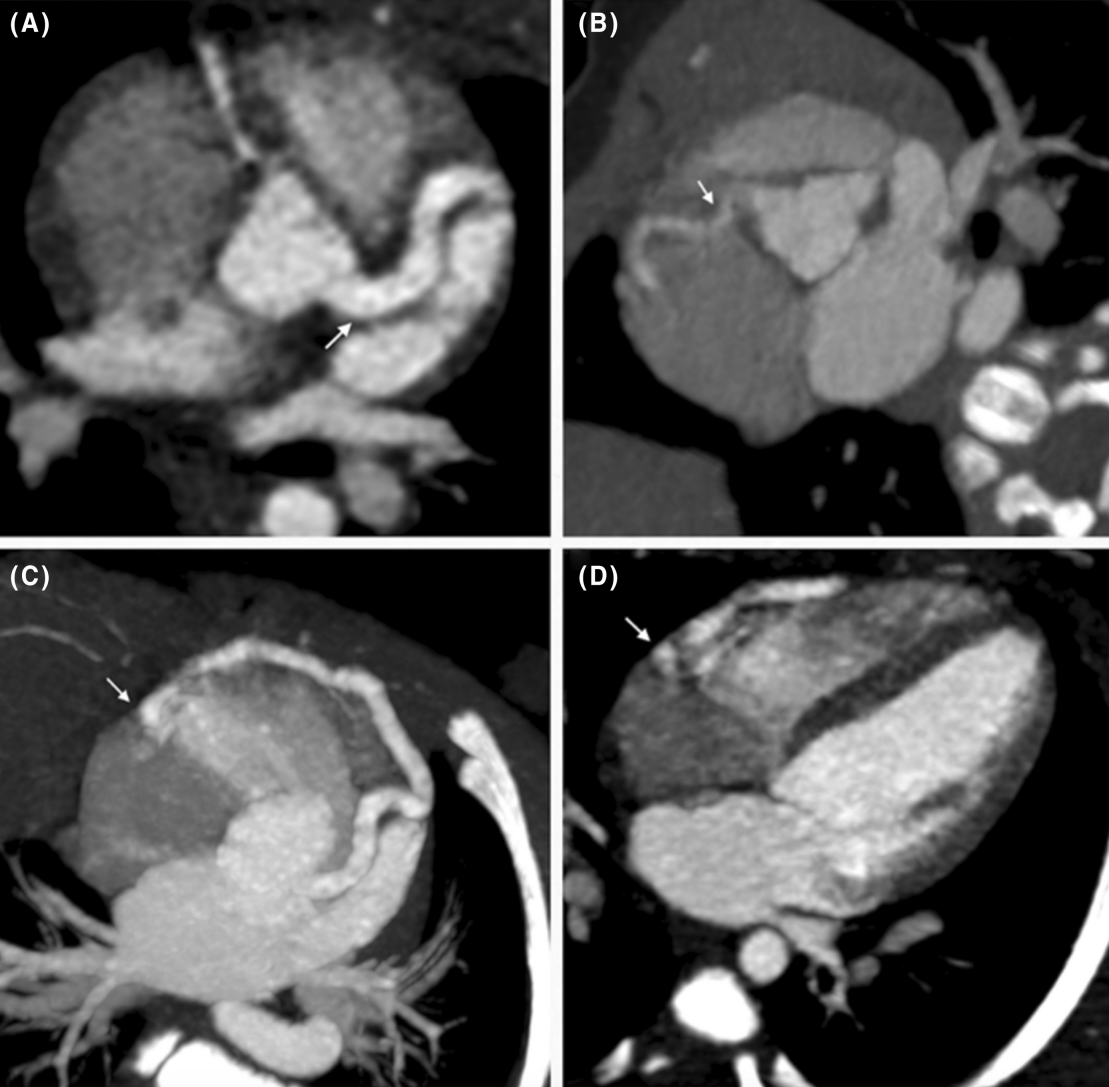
Coronary CT angiography findings: (A) ectasia of the left main coronary artery (4.7 mm, Z 10.9) and (C) proximal segment of the left anterior descending artery (3.9 mm, Z 10.2), where it forms a fistula that crosses the interventricular septum and the right ventricle (RV) free wall (arrow). (B) Proximal normal‐sized right coronary artery. (D) Four‐chamber view showing the coronary fistula drainage into the basal third RV free wall, a discernible opacification difference between the right cavities is also appreciated.
